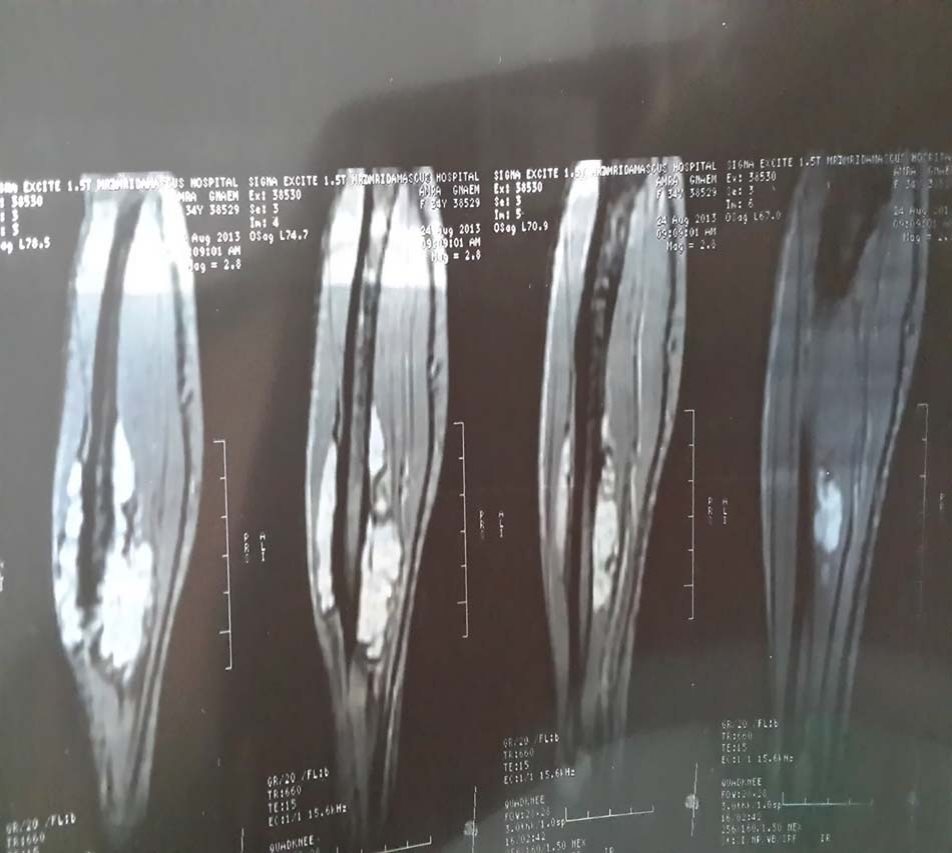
Showing the forearm MRI.
